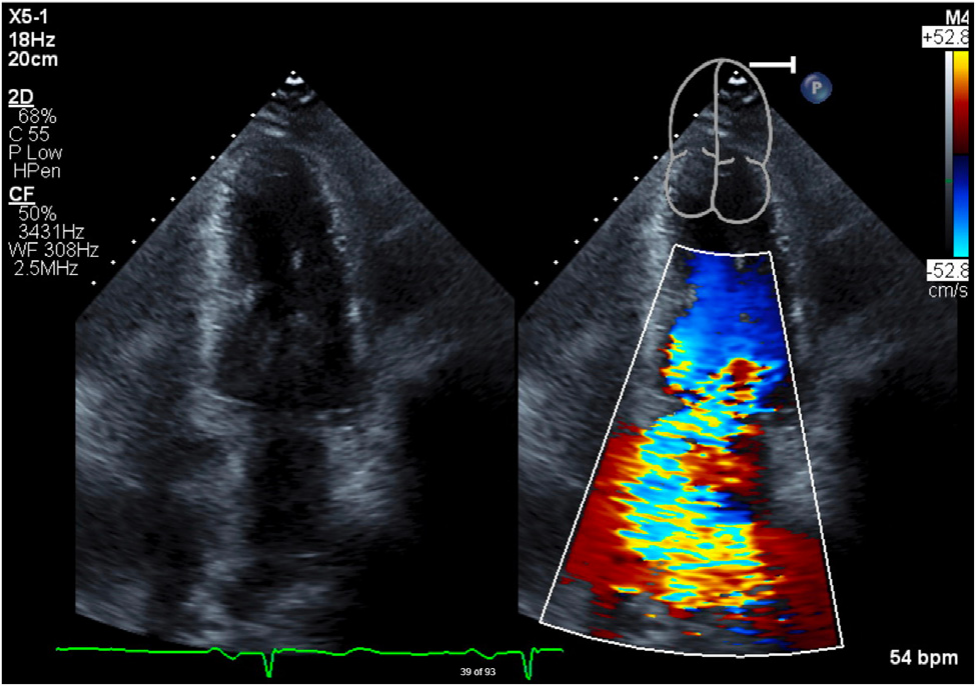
Two-dimensional TTE, apical four-chamber view, without (*left*) and with (*right*) color flow Doppler in systolic phase after administration of a UEA, demonstrates a large eccentric anteriorly directed MR jet.
